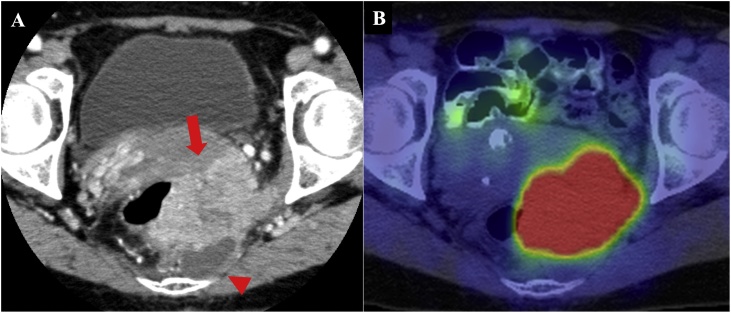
Contrast computed tomography reveals a 50-mm well-defined cystic mass in the presacrum (arrowhead) and a 70-mm solid mass extending from the cyst into the rectum, vagina, and left sciatic spine (arrow) (A). The solid mass demonstrates high fluorodeoxyglucose uptake on positron emission tomography (B).
